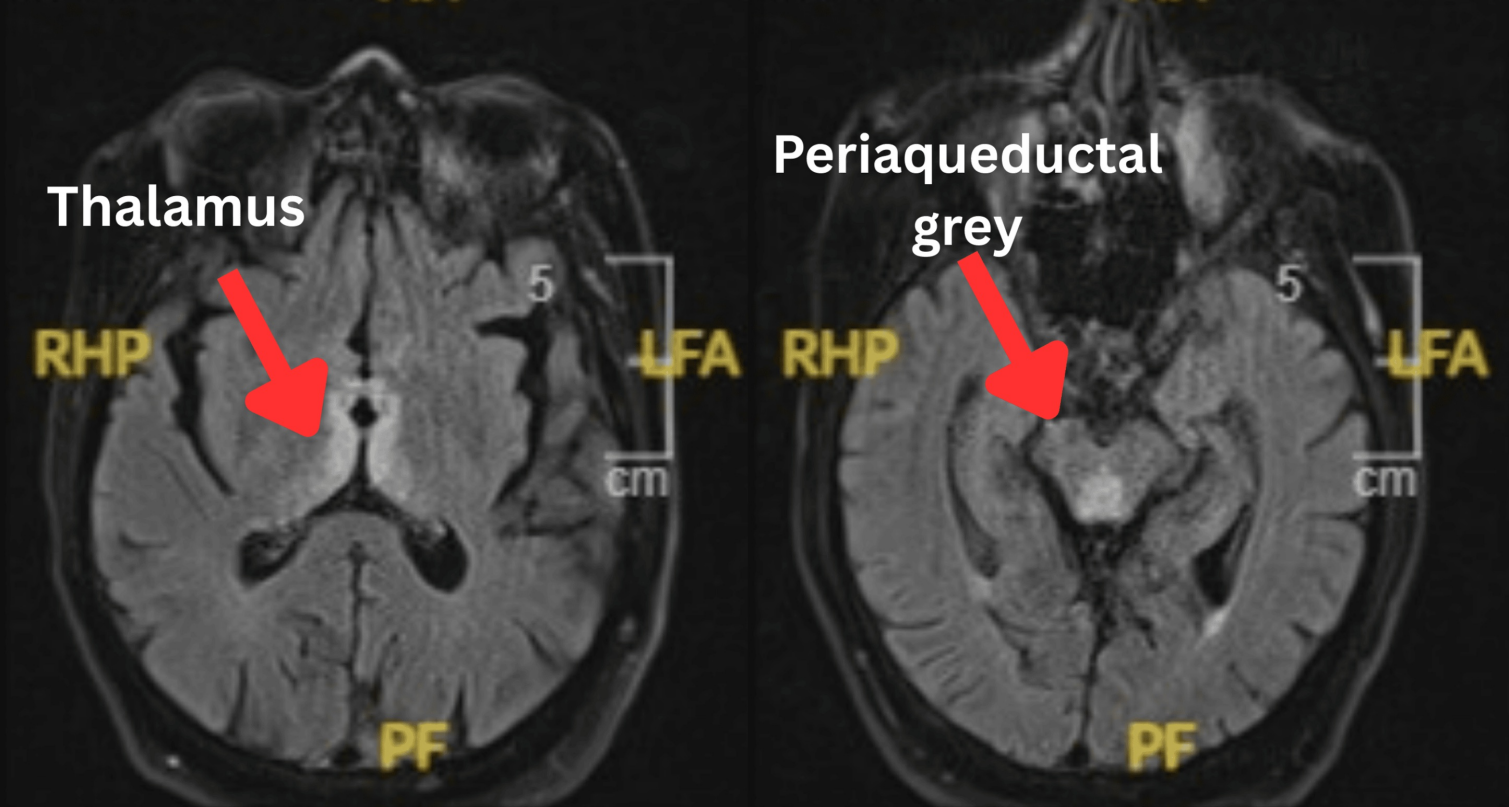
MRI brain (axial FLAIR) demonstrating symmetrical hyperintense signal involving the medial dorsal thalami, periaqueductal grey matter, tectal plate, and mammillary bodies, characteristics of acute WE. MRI: magnetic resonance imaging; FLAIR: fluid-attenuated inversion recovery; LFA: left frontal area; RHP: right hemispheric parietal; PF: posterior fossa; WE: Wernicke’s encephalopathy.
